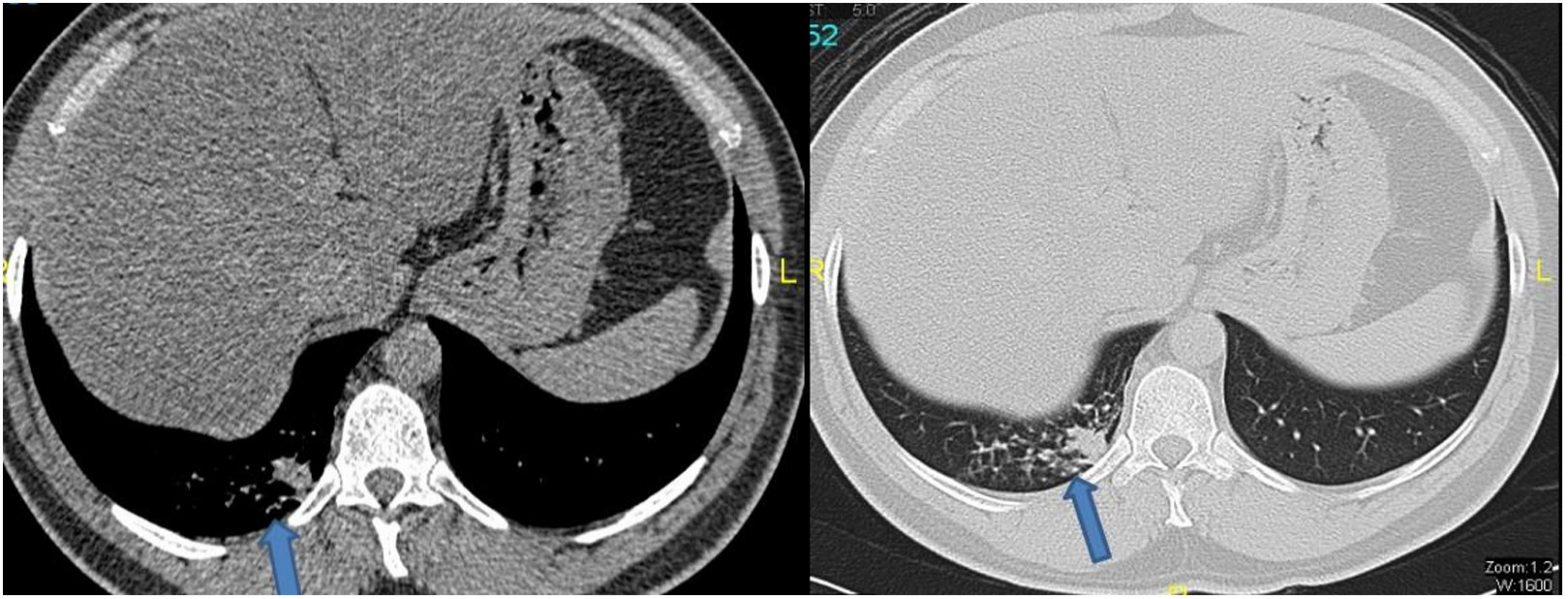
CT of the chest showing peripheral soft tissue density along the posterior basal segment of the right lung
